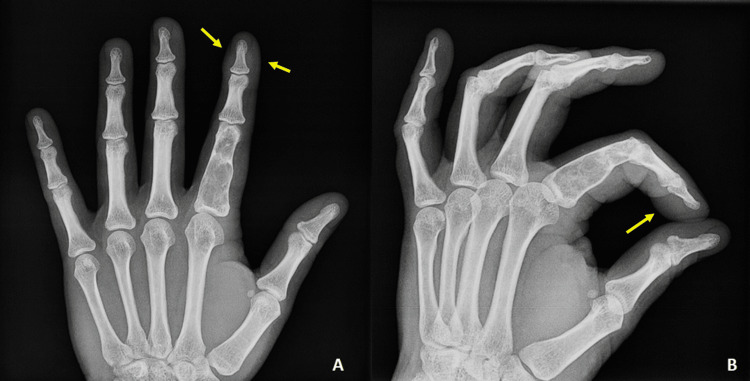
Face (A) and profile (B) x-rays of the left index finger. Enchondroma is obvious in both X-rays. The distal phalanx lump is nearly marked in both X-rays (arrows).
